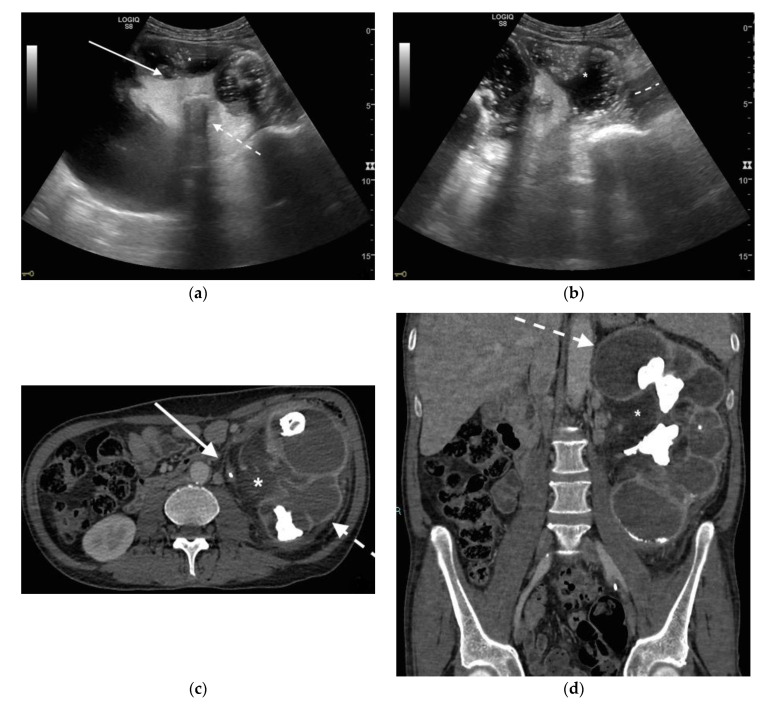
A 71 years old septic patient. US longitudinal (a,b) view of left kidney. Severe hydronephrosis, with extreme cortical thinning. High-grade dilatation and parietal thickening (white arrow) of calico-pelvic system fluid filled by inhomogeneous fluid with multiple hyperechogenic spots (*). Enlarged and markedly hyperechogenicity of renal sinus fat with acoustic shadowing due to staghorn calculi (dashed arrow). Perirenal fat inhomogeneity was detected (dashed line). Axial (c) and MPR coronal (d) CT with intravenous contrast in cortical-medullary phase after stent placement (white arrow), showing high-grade hydronephrosis with diffuse parietal thickening of calico-pelvic system (dashed arrow), staghorn calculi and renal sinus lipomatosis with mild inhomogeneous fat stranding and suffusion (*).
